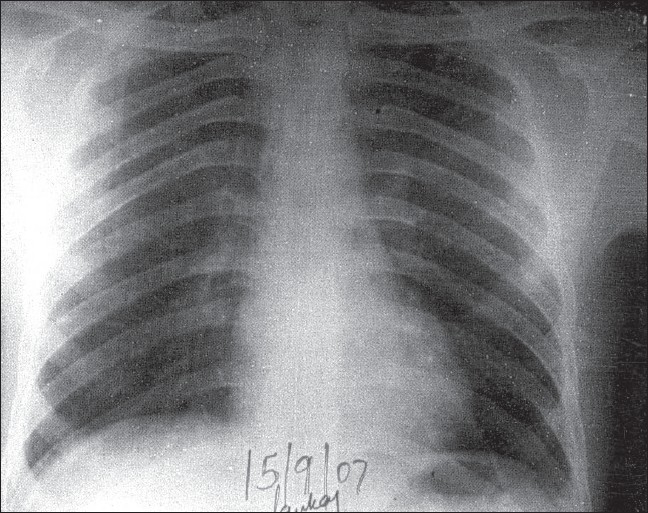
Chest radiograph done after four months is normal
